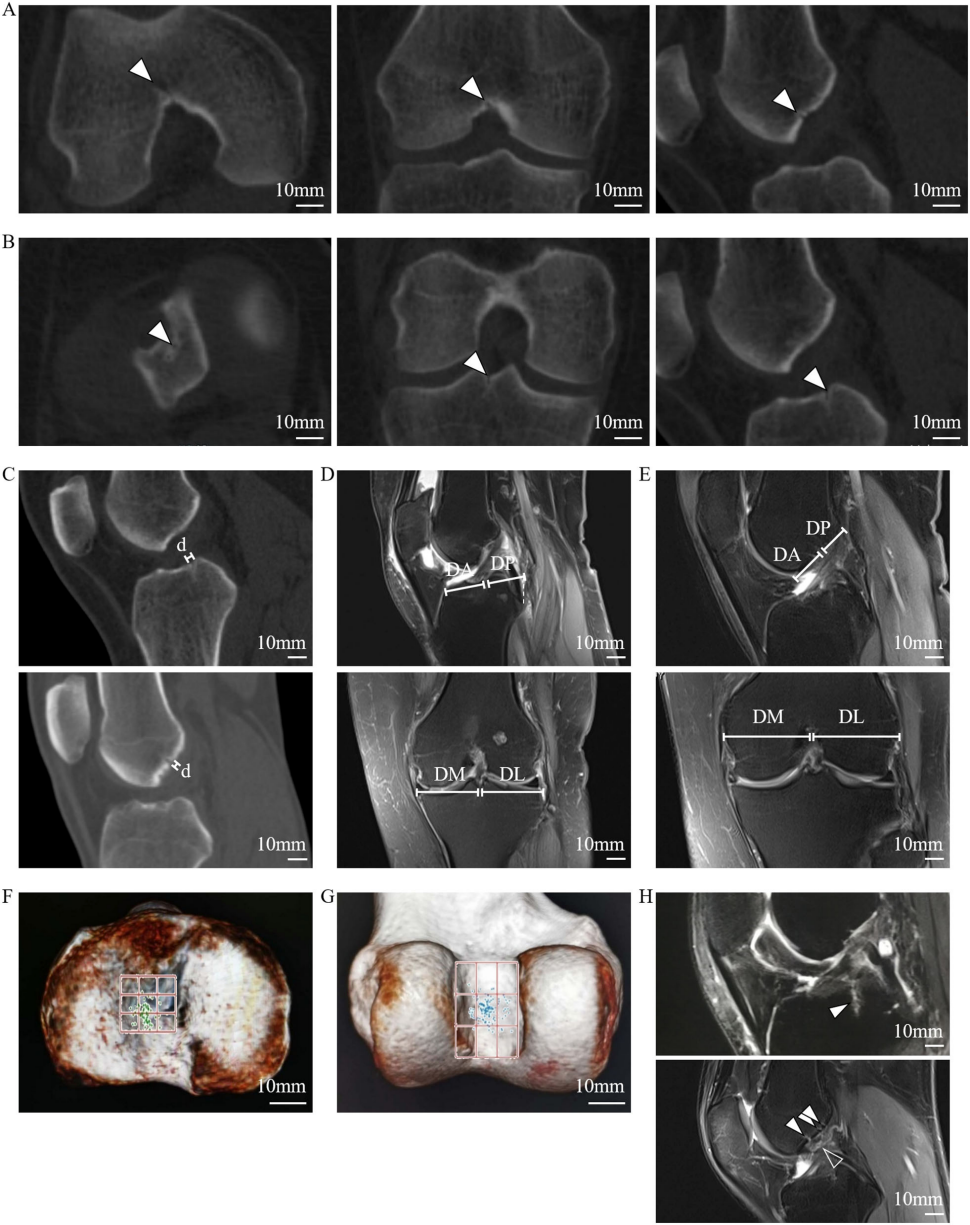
Radiographic features of the FTIE, IFFs, and enclosed tissues of the LC complex. (A) IFF (arrowheads) shown on 1-mm-thick reconstructed sagittal and coronal CT images. (B) FTIE (arrowheads) shown on 1-mm-thick reconstructed sagittal and coronal CT images. (C) Radiographic measurement of diameters of the FTIE and IFF shown as “d.” (D,E) Radiographic measurement of the DA, DP, DM, and DL of the FTIE (D) and IFF (E). (F) The distribution of FTIEs in the nine-zone model. (G) The distribution of IFFs in the nine-zone model. (H) The soft tissues (arrowheads) within the tibial and femoral LC complexes shown on MRI that appear to connect to the branches of the MGA or tributaries of the MGV (empty arrowhead).
